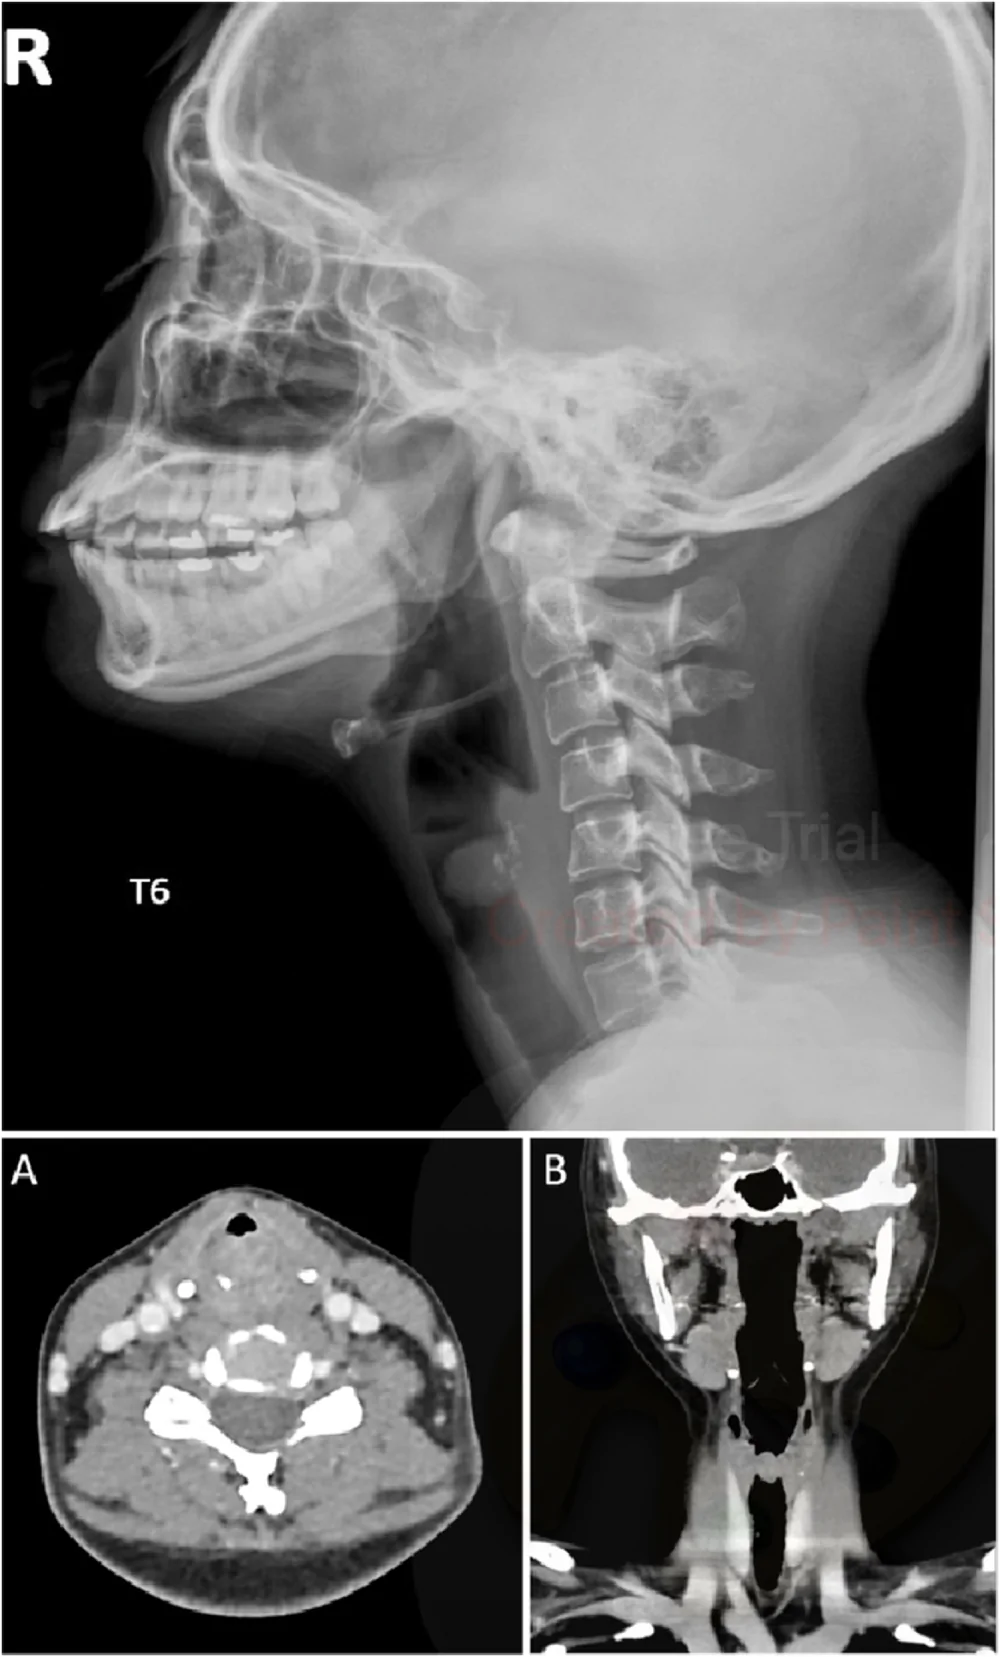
Top: Plain radiograph of the lateral neck showing a radiopaque mass attached to the posterior subglottic wall. Below: Computed tomography images showing a lobulated, infiltrative, enhancing mass at the posterior wall of the subglottis, causing severe narrowing of the laryngeal airway (A: axial view; B: coronal view).
